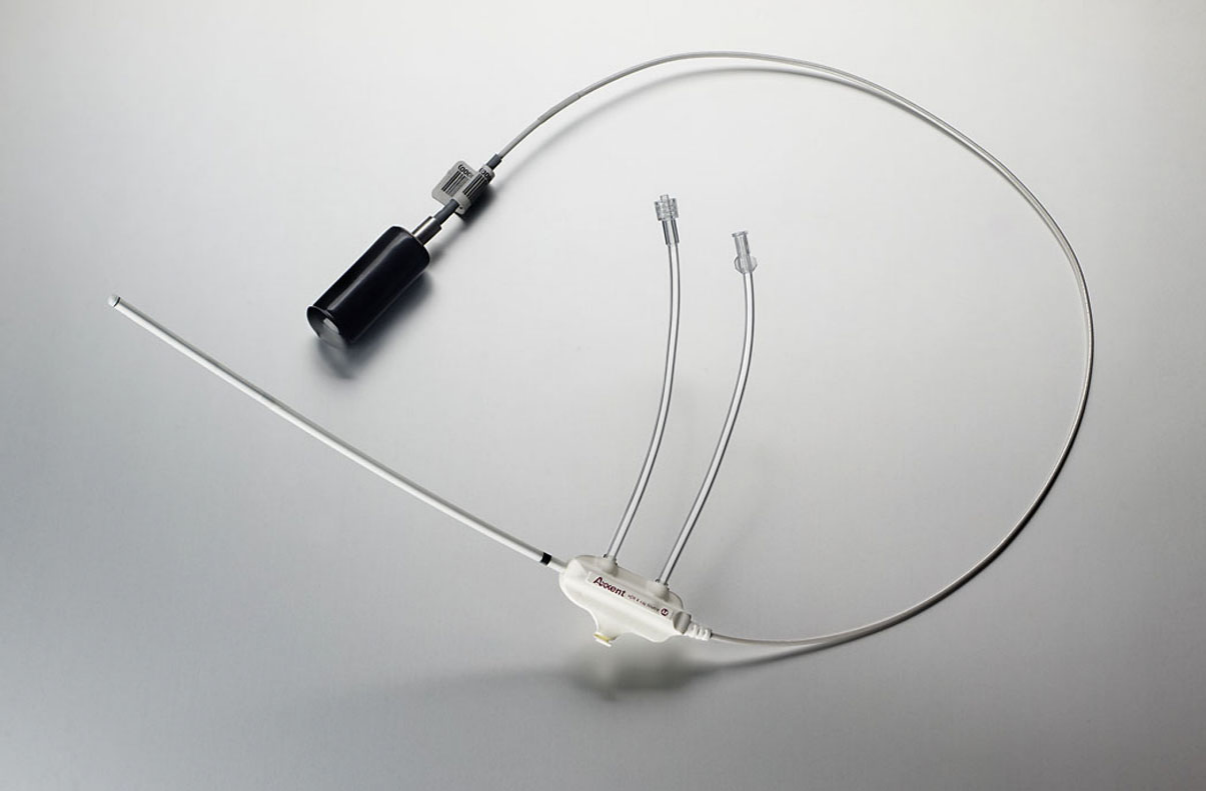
Axxent electronic brachytherapy, HDR X-ray source (courtesy of Xoft).
